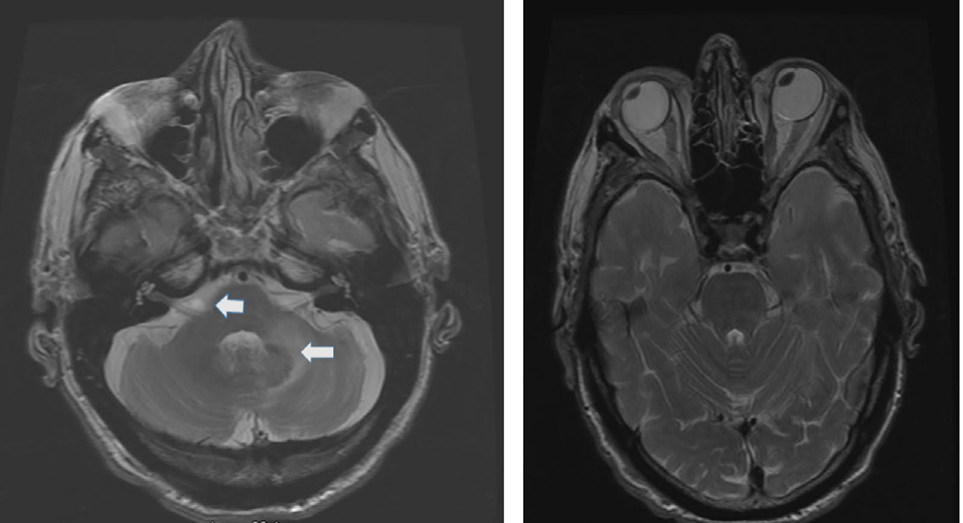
Axial T2 MRI: left panel shows a small area of increased signal intensity due to a small stroke involving the lateral pons, near the root entry of the right vestibular nerve (smaller arrow) and a larger stroke involving the left lateral pons and middle cerebellar peduncle (left panel larger arrow). Notice the conjugate ocular deviation of the eye to the right, coinciding with the slow phase of the nystagmus related to the second lacunar stroke in the right pons.
